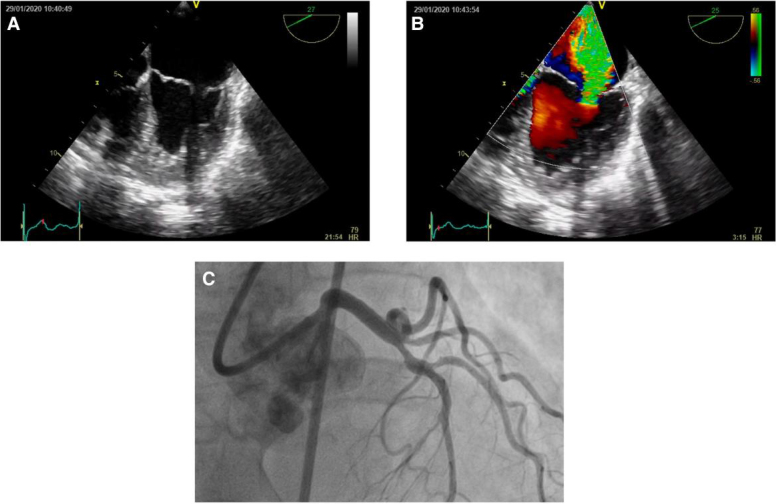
Initial transoesophageal echo and diagnostic coronary angiogram revealing severe mitral valve regurgitation. (*A*) 0–30 degrees four-chamber view in end-systole revealing coaptation gap of mitral valve leaflets. (*B*) Same image with colour revealing severe mitral regurgitation. (*C*) Diagnostic coronary angiogram right anterior oblique cranial view demonstrating 60–70% disease on proximal left anterior descending artery and 70% on ramus intermedius branch (small vessel).
